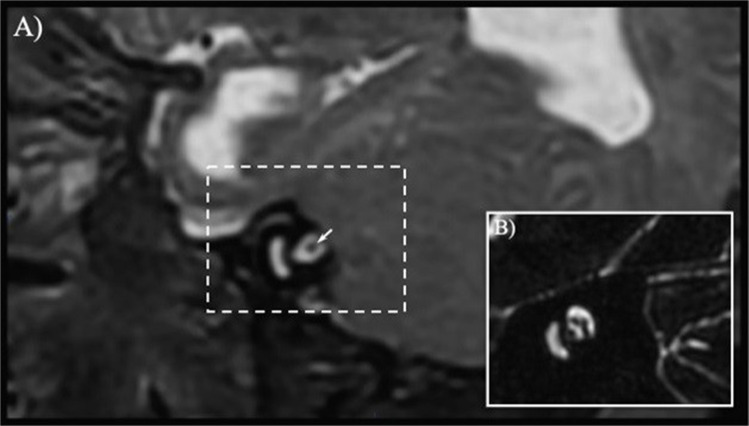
A Sagittal T2-weighted image in a child with Crouzon syndrome showing in the internal acoustic meatus a single dark dot (short white arrow; i.e., a single cranial nerve) presumably corresponding to the sole facial nerve due to vestibulocochlear nerve aplasia (or less likely to a fusion of seventh and eighth cranial nerves). B Sagittal T2-weighted image in a normal subject showing four dots in the internal acoustic meatus corresponding to the facial nerve and the cochlear, superior vestibular and inferior vestibular branches of the vestibulocochlear nerve. Partially reproduced from Valeggia et al. [73], with permission from Springer Nature (www.springernature.com)
